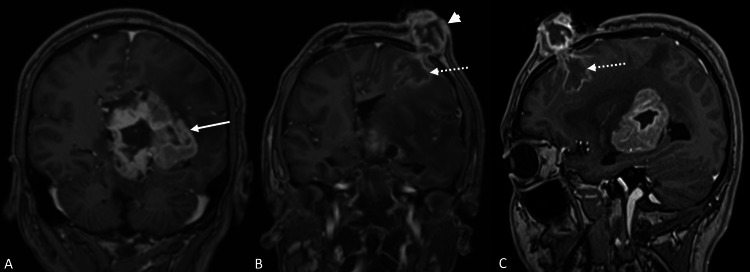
Postoperative T1-weighted contrast MRI taken two months after stereotactic biopsy and VPS (A) Tumor growth (solid arrow), with new growth along the biopsy track (broken arrows) continuous with the scalp (arrowhead) on (B) coronal and (C) sagittal views MRI, magnetic resonance imaging; VPS, ventriculoperitoneal shunt
